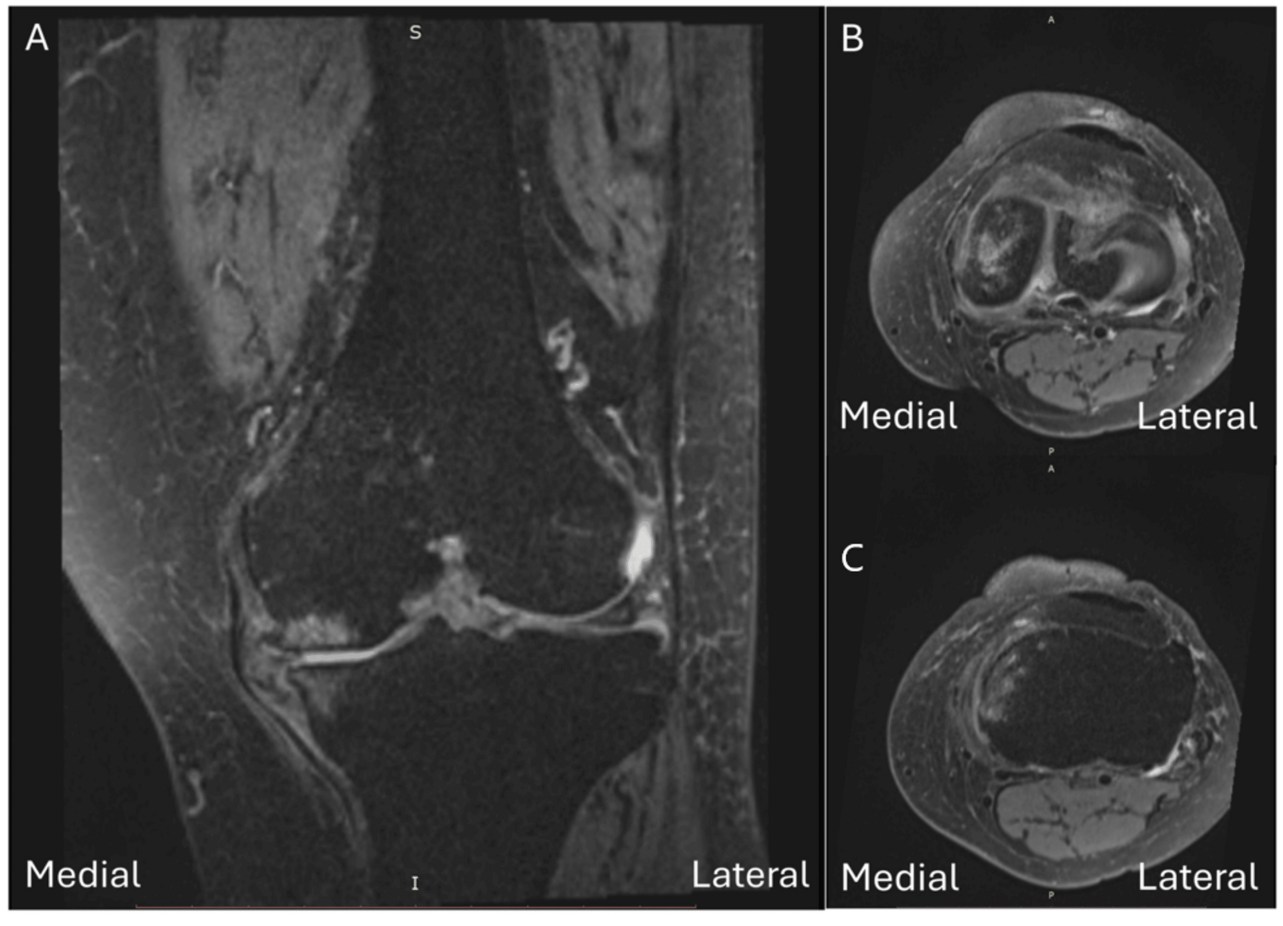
Magnetic resonance imaging (MRI) findings on proton density–weighted fat-suppressed fast spin echo (PDw FS FSE) sequences. (A) Coronal image shows high signal intensity in the medial femoral condyle and medial tibial plateau, consistent with bone marrow lesions, together with a grade 3 medial meniscus tear with extrusion. (B) Axial image shows high signal intensity in the medial femoral condyle. (C) Axial image shows high signal intensity in the medial tibial plateau.
